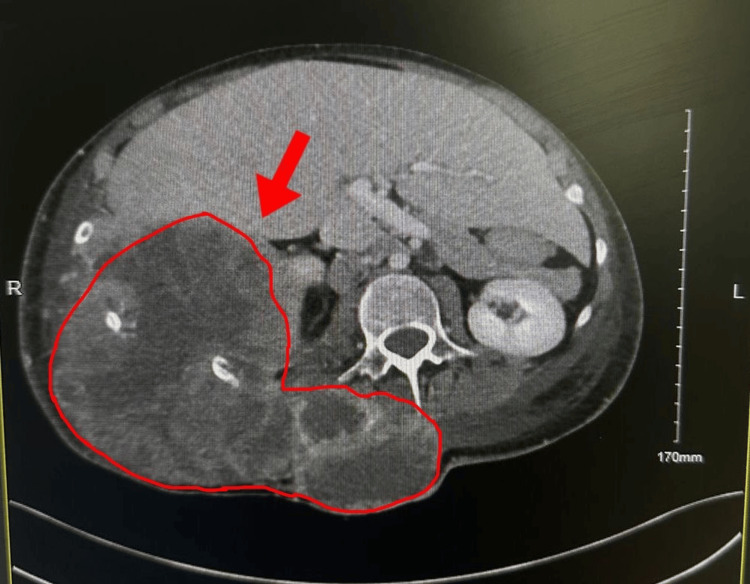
An abdominal CT image with inferior tumor extension abutting the liver and right kidney
